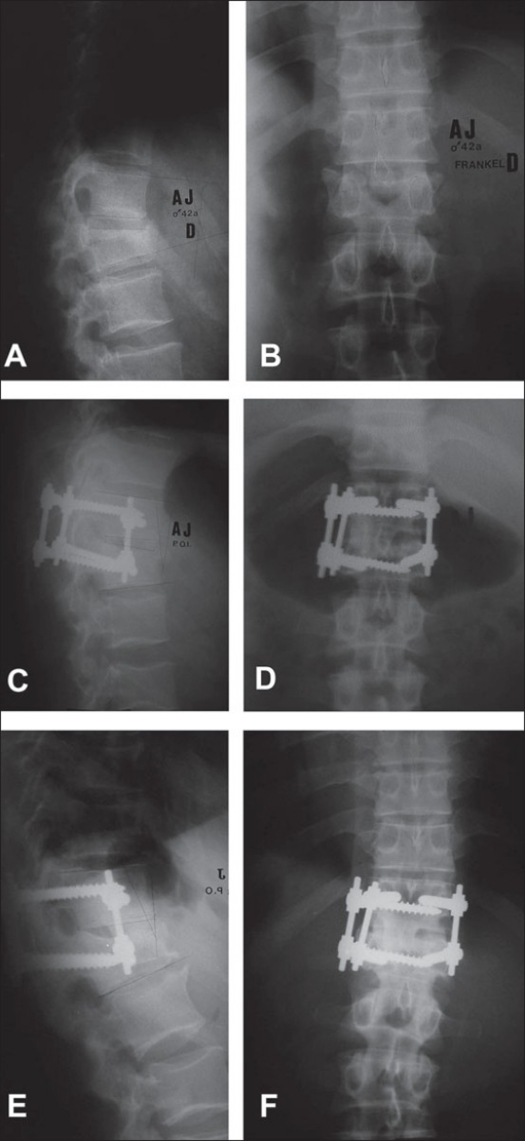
Preoperative X rays of dorsolumbar spine AP and lateral (A & B) show a lumbar fracture of type A. Immediate postoperative X ray (C & D) and after 13 years radiographic follow-up (E & F) show fracture consolidation after monosegmental fixation with a combined approach
